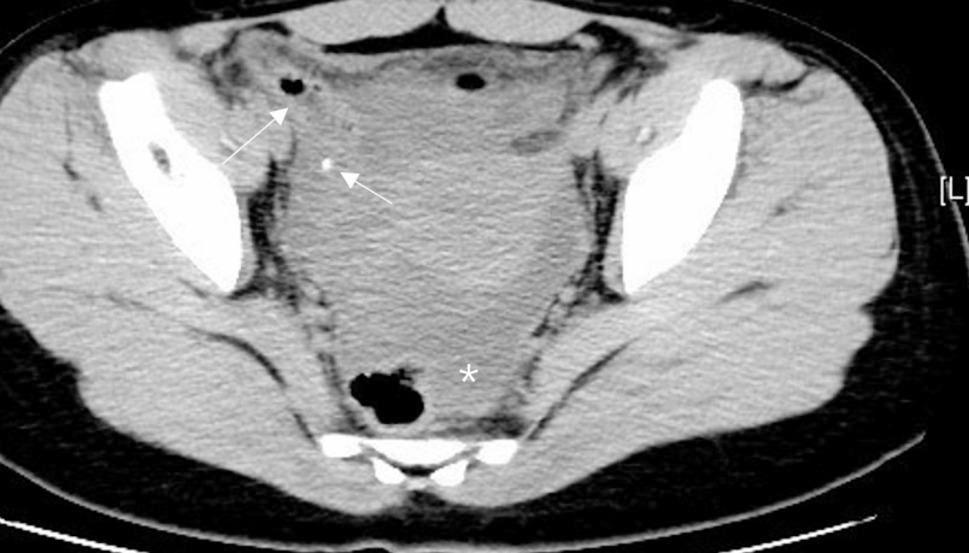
Extraluminal appendicolith and fluid. Axial CT images show an enlarged appendix with periappendiceal appendicolith (arrow) and large periappendiceal fluid (asterisk)
